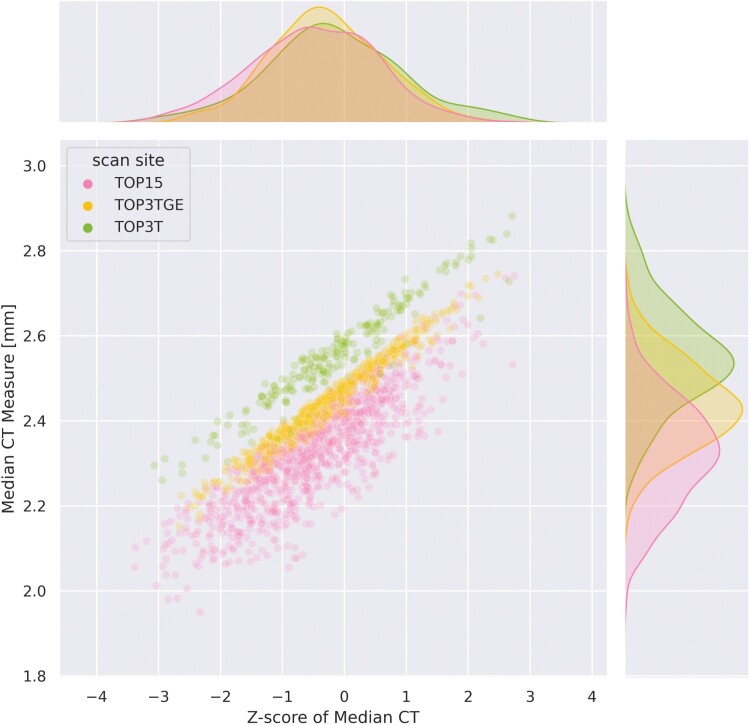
Joint-plot and marginal distributions of the median CT measures (*y*-axis) and associated deviation scores (*x*-axis), color-coded by a scanner, from the test samples of the adaptation set (cross-sectional TOP samples).
